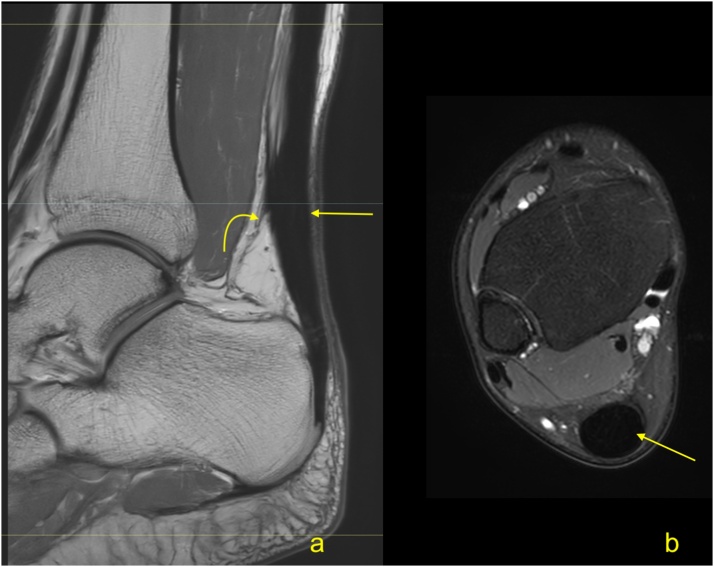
A 63-year-old patient who underwent a partial Achilles tendon rupture about 8 months ago, with an excellent clinical outcome. Remodeling of the Achilles tendon (straight arrow) and adhesions (curved arrow) are visible on MRI. A- PD-weighted, b- PD-weighted with fat suppression.
